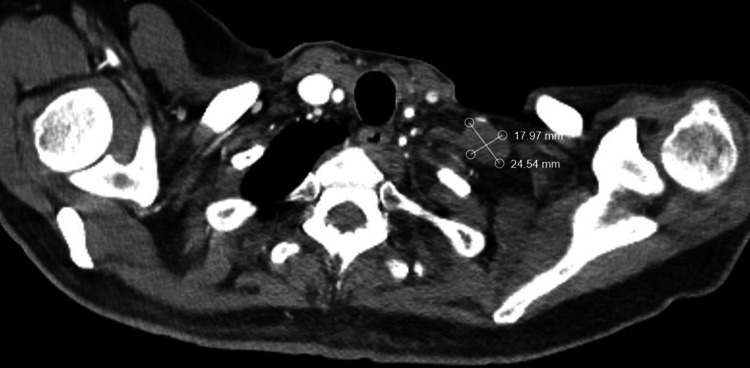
Latest surveillance axial computed tomography (CT) images of the chest Axial CT imaging scans with IV contrast re-demonstrating a stable left lower cervical 15 months after completion of radiation therapy. Measurements of residual disease demonstrate no significant progression by RECIST v1.1 criteria. RECIST: Response Evaluation Criteria in Solid Tumours
